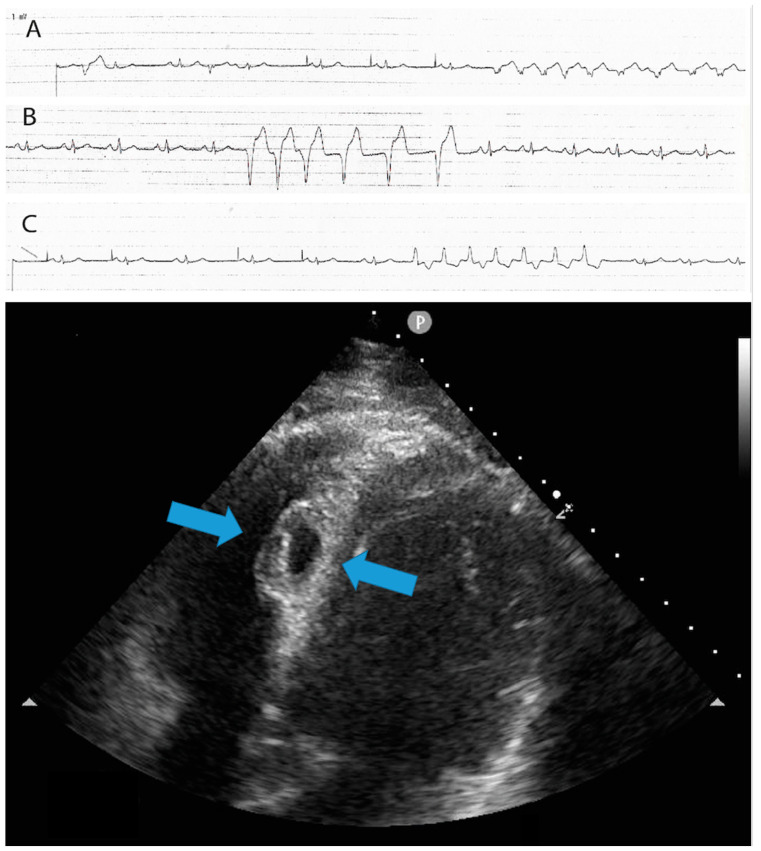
The upper part of the image shows the presence of non-sustained ventricular tachycardia (NSVT) and ventricular ectopic beats of different morphologies, which developed at the end of the procedure and persisted the following day. The lower part of the image displays a 4-chamber view echocardiogram conducted the day after the procedure. It reveals the presence of an interventricular septal hematoma measuring 17 × 10 mm, which protruded into the right ventricle (RV)—light blue arrows. A, B and C strips shows non-sustained ventricular tachycardia with different morphologies.
